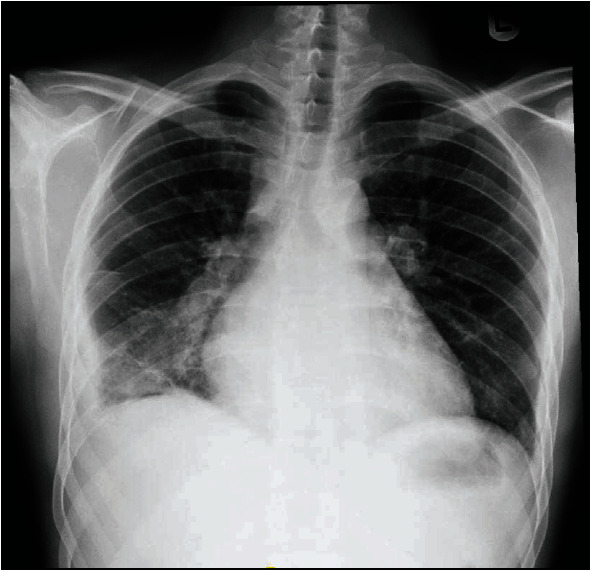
Patient's chest X-ray demonstrating cardiomegaly and right lower lobe consolidations with pleural effusion.
